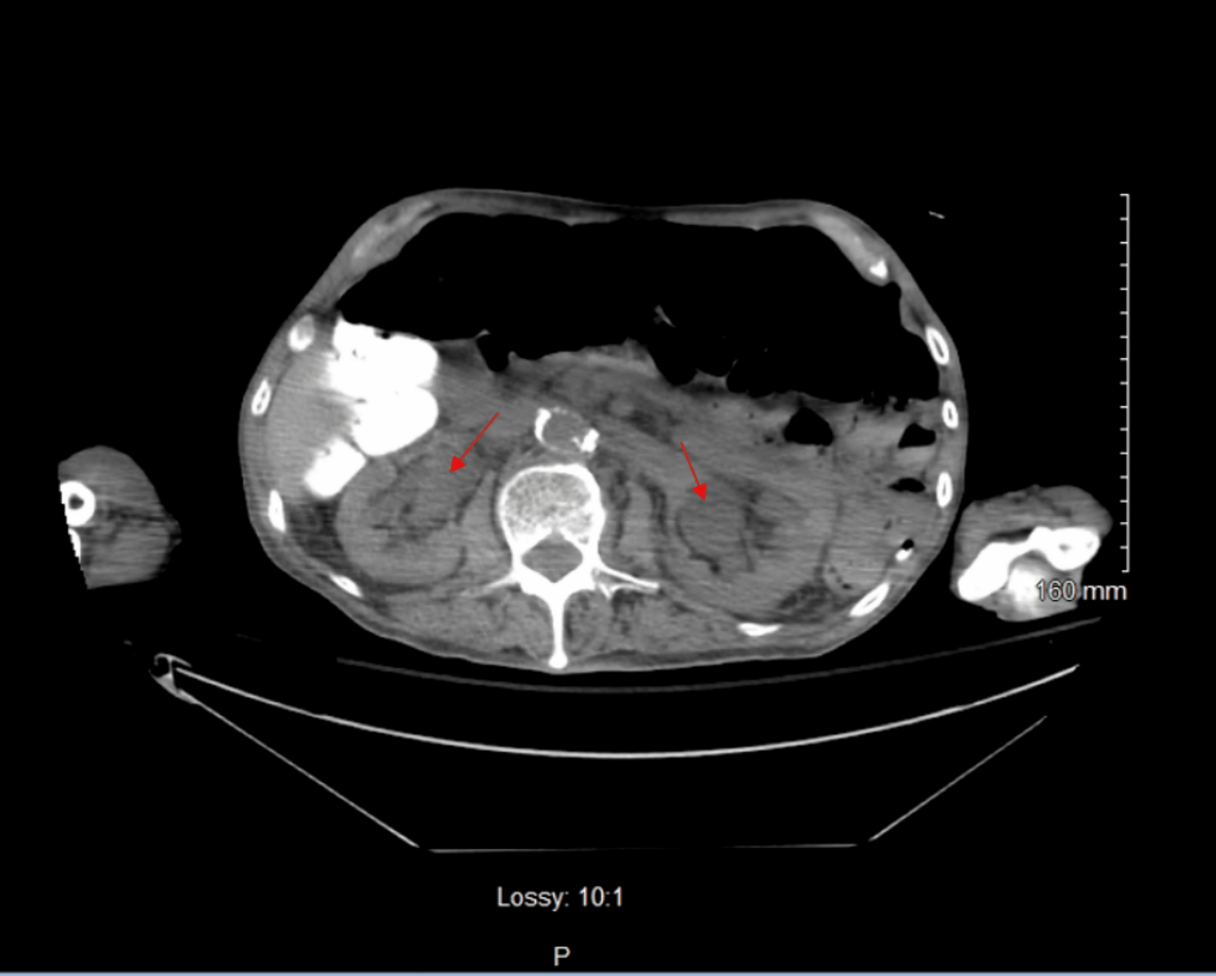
Axial non-contrast CT image at the renal level Mild-to-moderate bilateral hydroureteronephrosis, with red arrows pointing to both sides.
